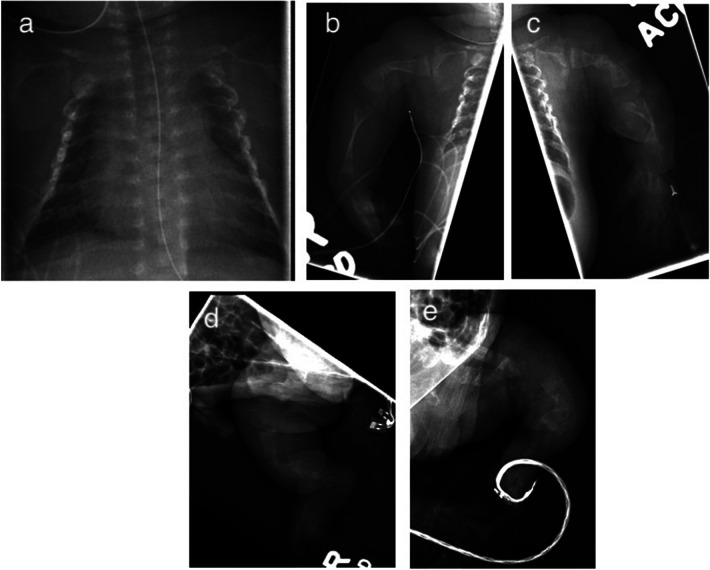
Initial x-ray demonstrating multiple bilateral rib fractures (a) and long bones (b–e) with curvilinear configuration and irregularities consistent with various stages of healing.
